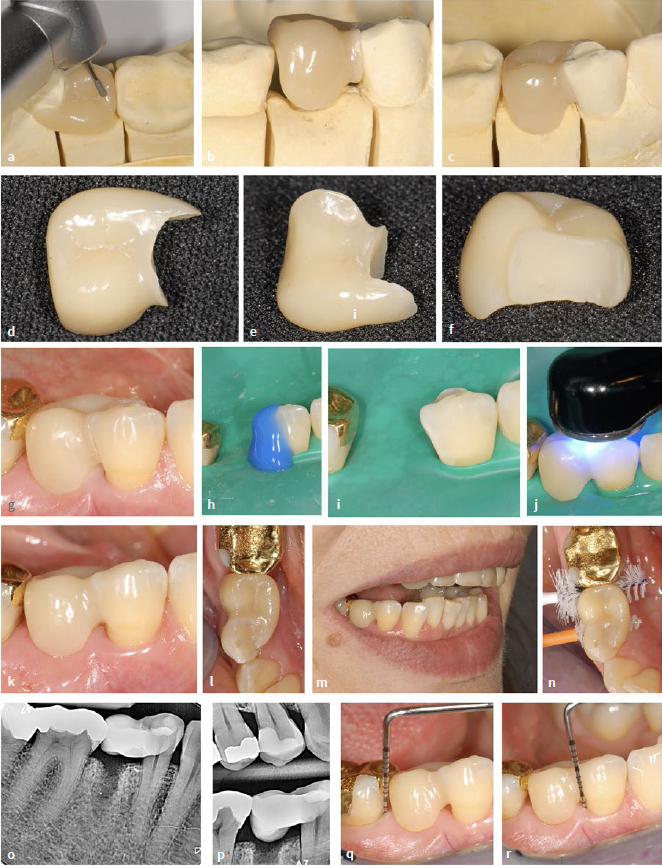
Same patient as in Fig 6. (a) Finishing and polishing on the model. (b) and (c) The distal surface of the pontic is shaped so that the workpiece can be “snapped” into place with the clearest possible position. (d) and (e) Finished and polished workpiece. (f) After blasting the adhesive surface with aluminum oxide powder. (g) Try-in on the patient. If positioning is not clear, an individual fixing splint can be used (see Fig 9o). (h) to (j) Etching, rinsing and drying of the abutment tooth under rubber-dam and adhesive placement with subsequent light curing. (k) After adhesive placement. (l) Note the selective oversizing in the area of the oral surface of tooth 44. (m) Overview. (n) Fitting of suitable interdental brushes. (o) and (p) Radiographic control. (q) and (r) Control after 4 months, no pathological findings, disappearance of bleeding on probing.
